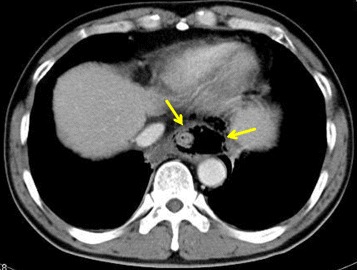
A chest CT scan revealed pneumomediastinum
